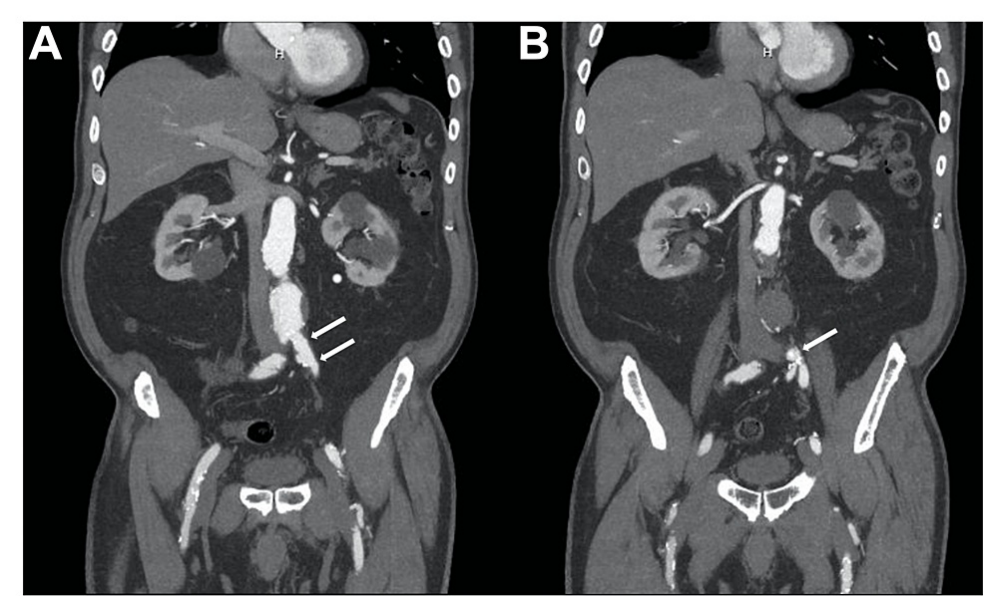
Short left common iliac artery: coronal pre-procedural computed tomography angiography coronal slices showing the left common iliac artery (panel A) and bifurcation (panel B). Note the short left common iliac artery of less than 1 cm in length (white arrows).
